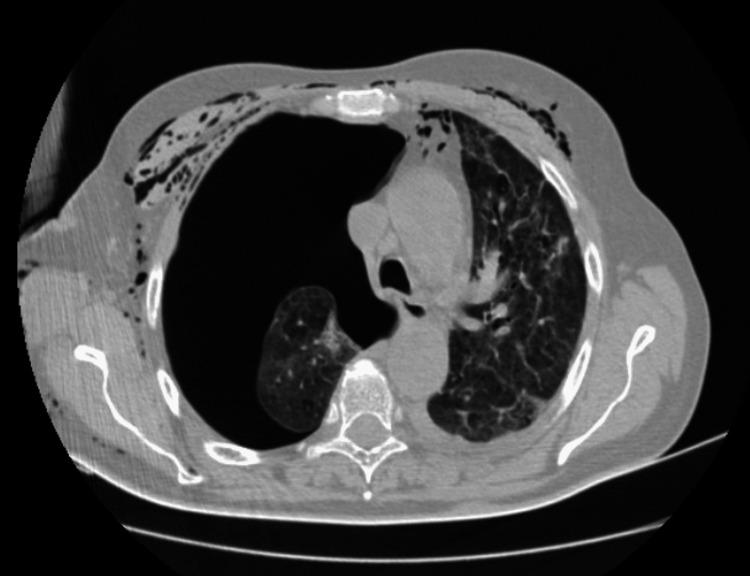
Contrast-enhanced chest CT (4th day of tube thoracostomy) showing no full expansion of the right lung, subcutaneous emphysema, and bilateral areas of small nodular and linear opacities.
